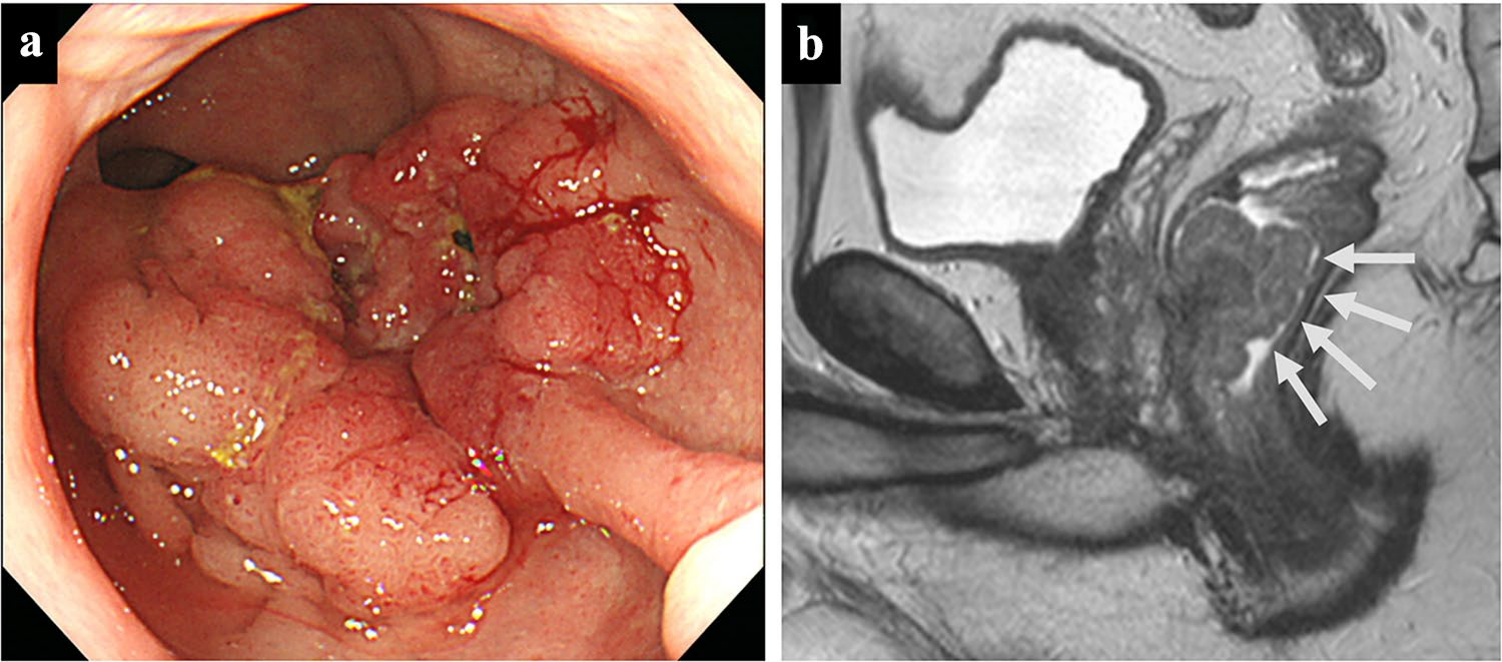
Colonoscopy and MRI at before the surgical treatment of rectal cancer. a Colonoscopy revealed a half circular 40-mm type 2 tumor in the anterior wall of the lower rectum. b MRI (T2WI: sagittal) revealed an irregular mass on the anterior wall side of the lower rectum; prostate invasion was suspected (arrows)
